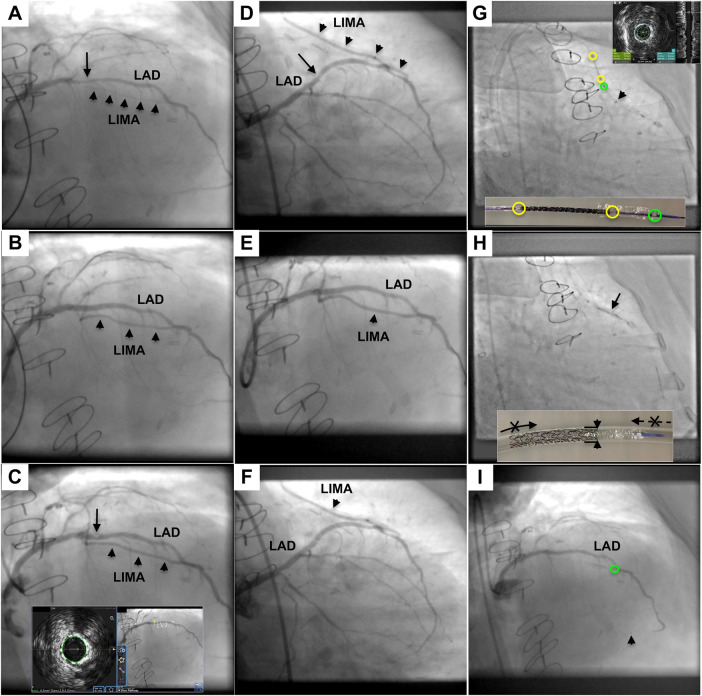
Deliberate occlusion of a dysfunctional LIMA graft using the “condom technique,” conventional angiographic images: (A) high-grade stenosis (arrow) of a wrap-around left anterior descending (LAD) artery. The “string phenomenon” (arrowheads) of the left internal mammary artery (LIMA) graft is also shown. (B) Good results after drug-eluting stent-assisted angioplasty of the LAD artery. Note the reverse flow through the dilated LIMA graft (arrowheads). (C) In-stent restenosis (ISR; arrow) of the LAD artery at the site of a well-expanded stent, showing an intravascular ultrasound (IVUS)-derived minimal stent area of 6.5 mm^2^ (embedded panel). Reverse LIMA graft flow (arrowheads) is also shown. (D) ISR (arrow) of the LAD artery and reverse LIMA graft flow (arrowheads) shown in the right anterior oblique (RAO) caudal view. (E) and (F) Good results after treatment of the ISR by drug-coated balloon angioplasty and reverse LIMA graft flow (arrowhead) depicted in RAO cranial and RAO caudal views, respectively. (G) Delivery of the assembly of a cut remnant of the distal part of a 2.25 mm angioplasty balloon mounted on a 2.25 mm stent (embedded bottom panel; the assembly is constructed by advancing the stent as distally as possible inside the balloon remnant, and then the balloon is brought back over the stent) in the LIMA graft through a guideliner catheter (arrowhead) to perform the “condom technique.” The length of the stent is indicated by the two dots of markers seen on both ends of the stent delivery balloon (yellow circles), while the distal end of the remnant of the angioplasty balloon is indicated by the green circle. Note that the dysfunctional LIMA graft was atheromatous (embedded IVUS image). (H) Stent deployment (arrow) proximal to the distal LIMA graft anastomosis, fixing the proximal part of the balloon remnant (embedded bottom panel; black lines) on the LIMA graft wall. This way, a dead end is created at the stent–balloon interface (arrowheads), preventing retrograde blood flow from the native coronary artery to the corresponding graft (dashed arrow). Also, the fixed balloon remnant hanging in the graft lumen represents another dead end preventing blood flow from the graft to the corresponding native coronary artery (solid arrow). (I) Absence of a retrograde flow from the LAD artery to the LIMA graft, indicating successful occlusion of the graft. The distal end of the remnant of the angioplasty balloon, whose proximal part became trapped between the stent and graft wall, thereby forming a dead end, is indicated by the dot of the marker marked with a green circle. The dot of the marker marked with an arrowhead represents the distal end of the remnant balloon, which dislodged from the assembly during the first attempt to perform the “condom technique”; it was deliberately wedged in the distal part of the wrap-around LAD artery, causing occlusion.
